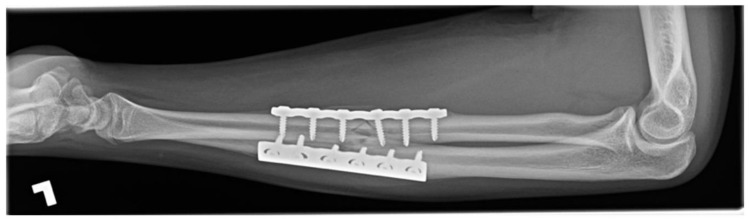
Twelve-week radiograph of an operatively treated both-bones forearm fracture in a pediatric electronic cigarette user. The ulna is healed, but the radius is not.
